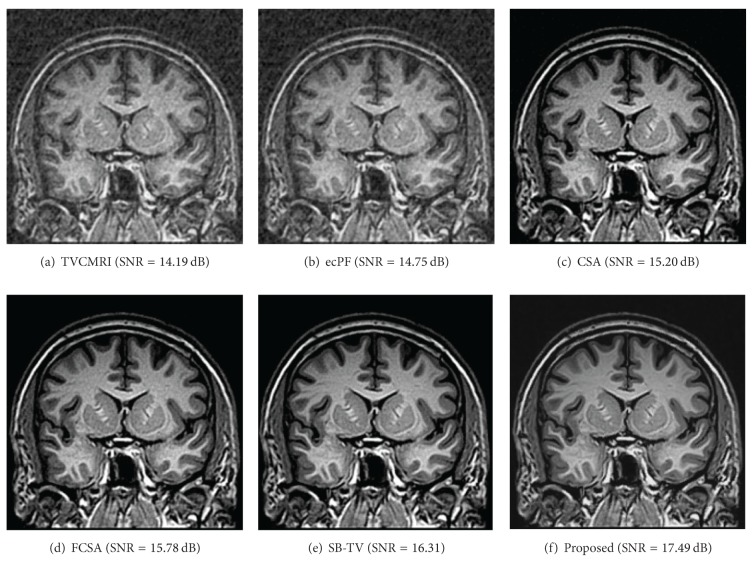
Brain MR image reconstruction from 20% samples (Gaussian noise case).
